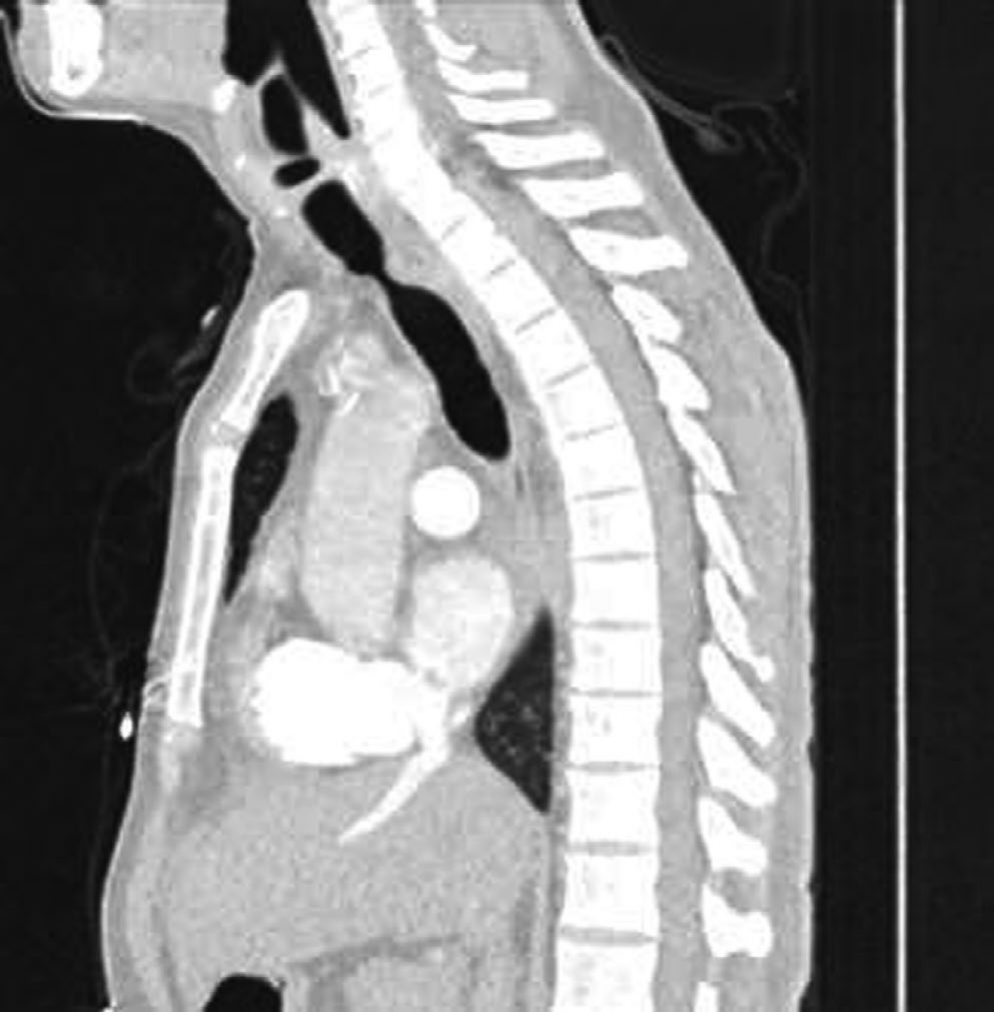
Sagittal section of the CT‐scan Chest showing the significant narrowing of the tracheal at the second thoracic vertebral level
